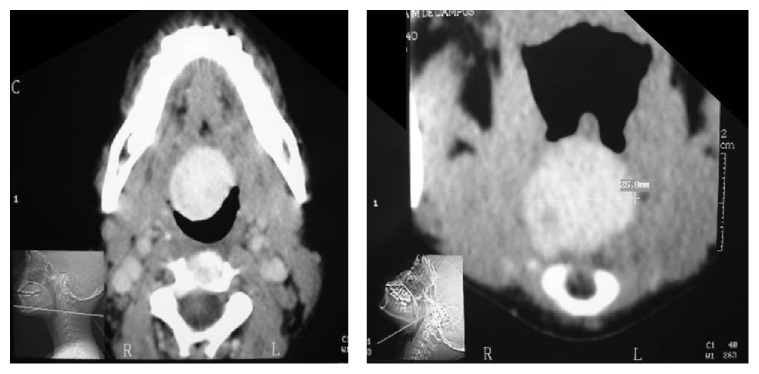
CT scan shows in axial cut a well defined hyperdense image of approximately 25.8 mm at its largest diameter, with infiltrative growth in the posterior region of the base of the tongue.
